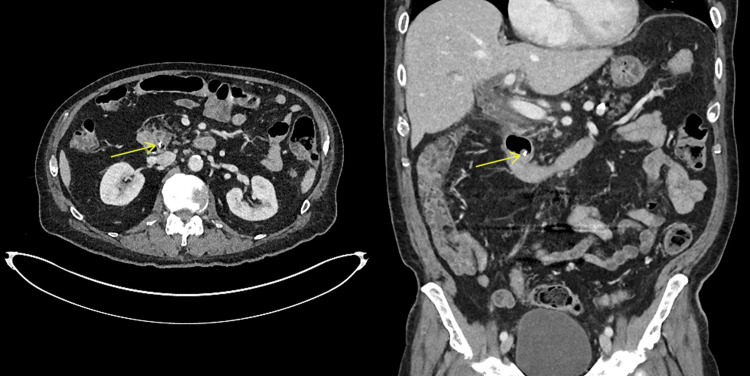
Axial and coronal CT images from the patient's third presentation to the hospital showing the migrated surgical clip in the distal common bile duct causing biliary obstruction.
